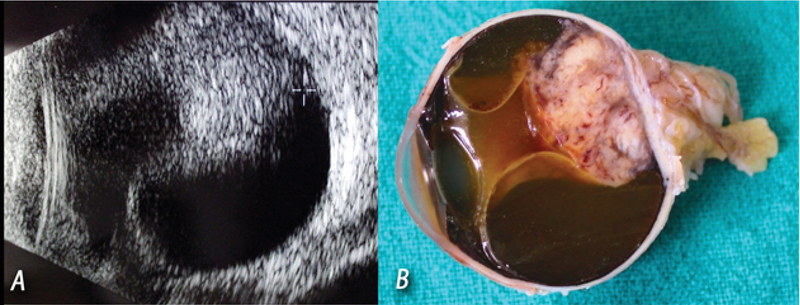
(A) Ocular ultrasound demonstrating a heterogeneous intraocular choroidal tumor associated with retinal detachment. (B) Enucleated surgical tissue from the eye with a whitish tumor mass with signs of hemorrhage.
